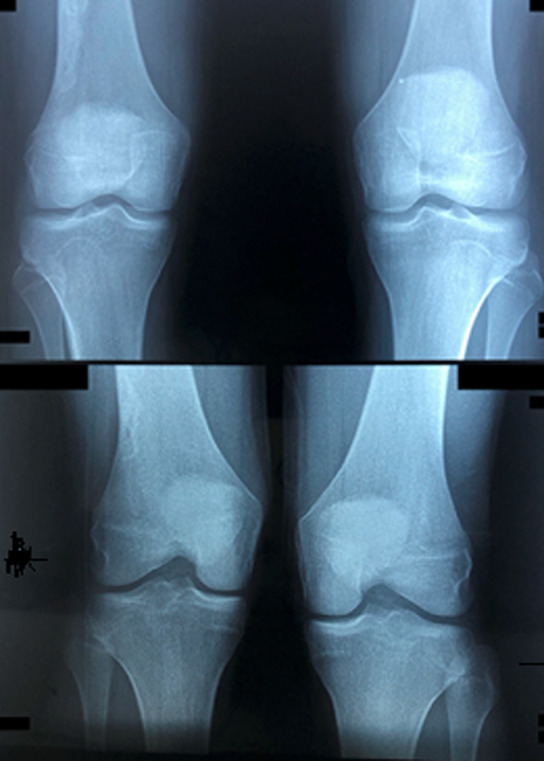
radiographie standard des genoux (de face)
